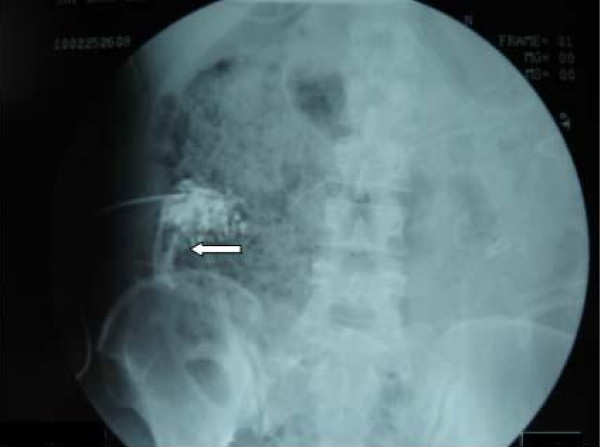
Abdominal radiography of this patient. Meglumine Diatrizoate was injected via the right drainage catheter (left arrow). The duodenum did not show up after injection.
